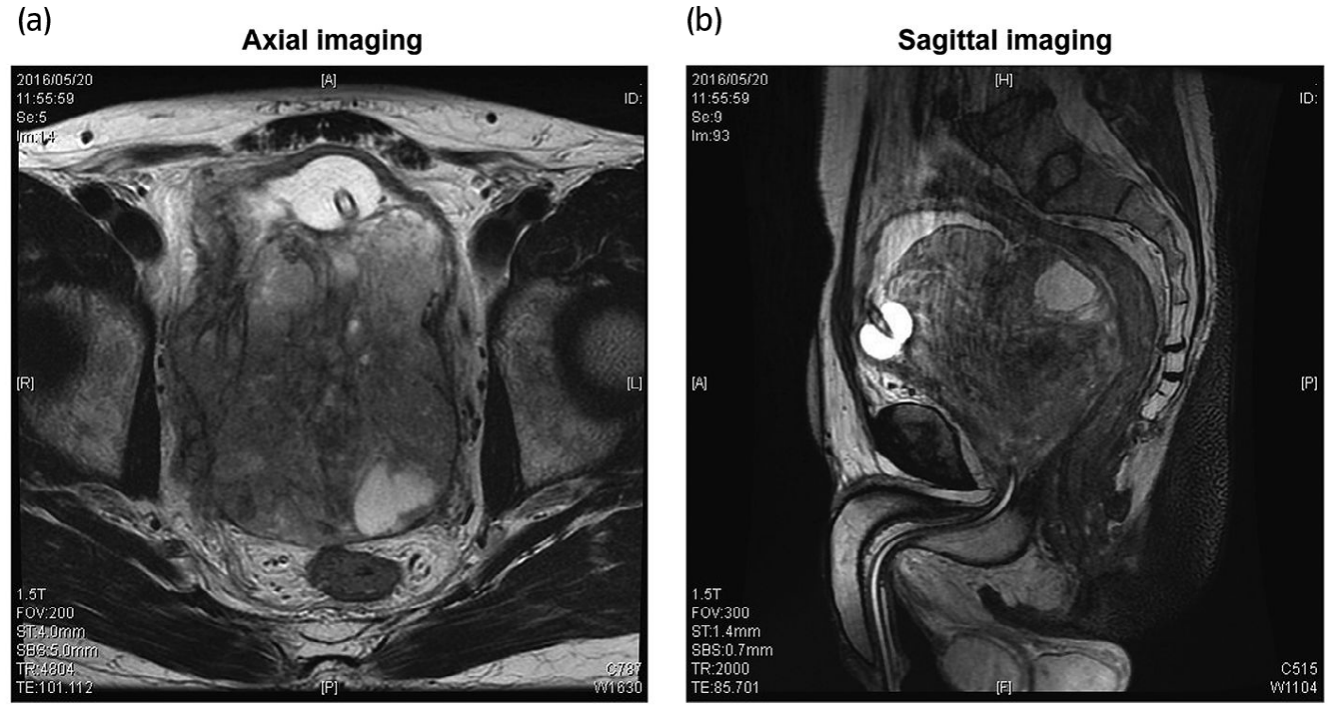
MRI (T2‐weighted image). (a) Axial view, (b) Sagittal view. MRI showed a whole enlargement of prostate tumor (10.6 × 9.3 cm) with heterogeneous signals. The prostate tumor protruded into the urinary bladder and invaded the bilateral seminal vesicles, but the rectal wall and pelvic lateral walls were not invaded.
